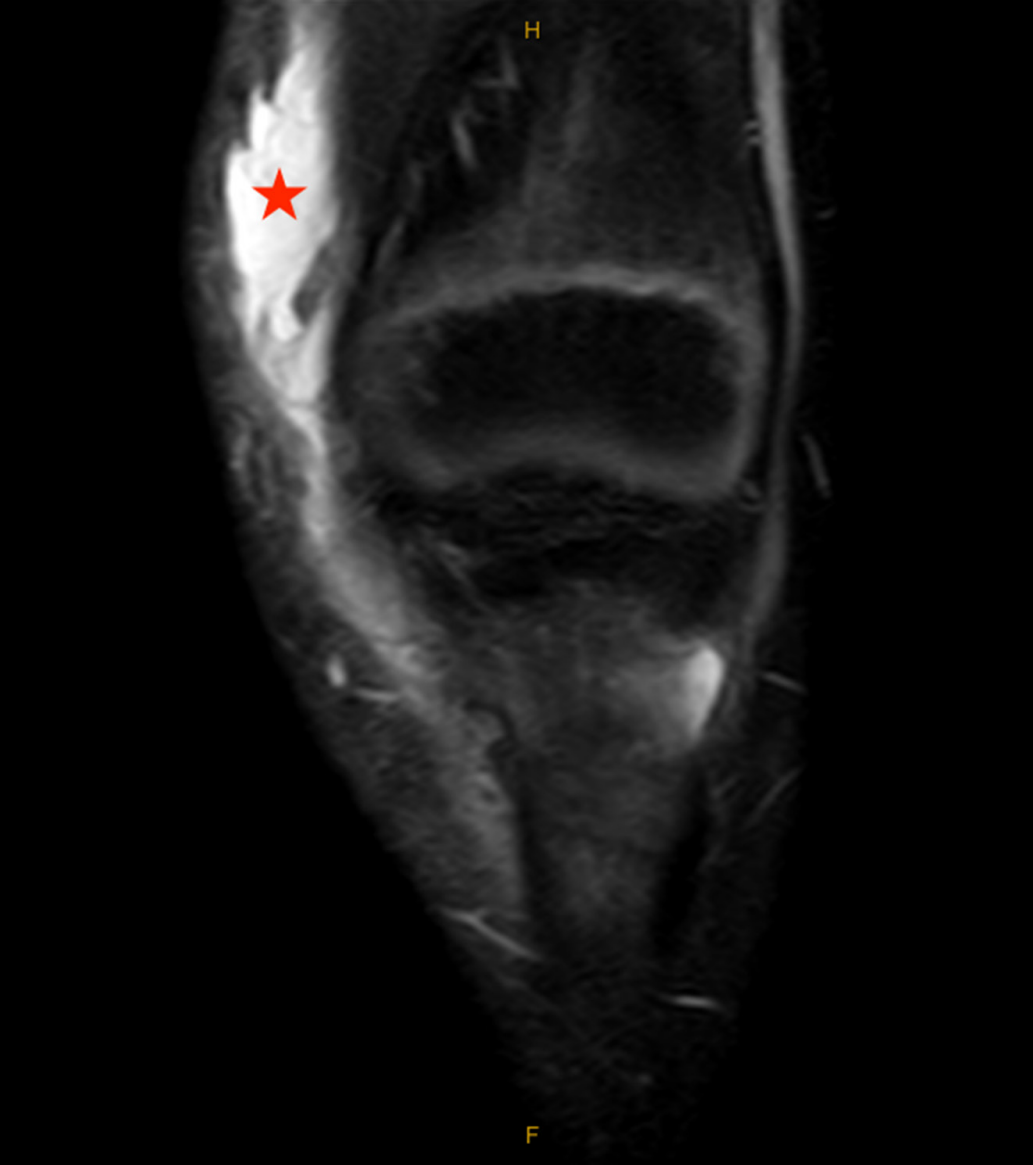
Coronal MRI; Proton density fat‐saturation coronal MRI sequence of the left knee obtained 8 days after the initial injury showing a subcutaneous fluid collection (star) at the anteromedial aspect of the knee, measuring about 5 cm craniocaudal.
